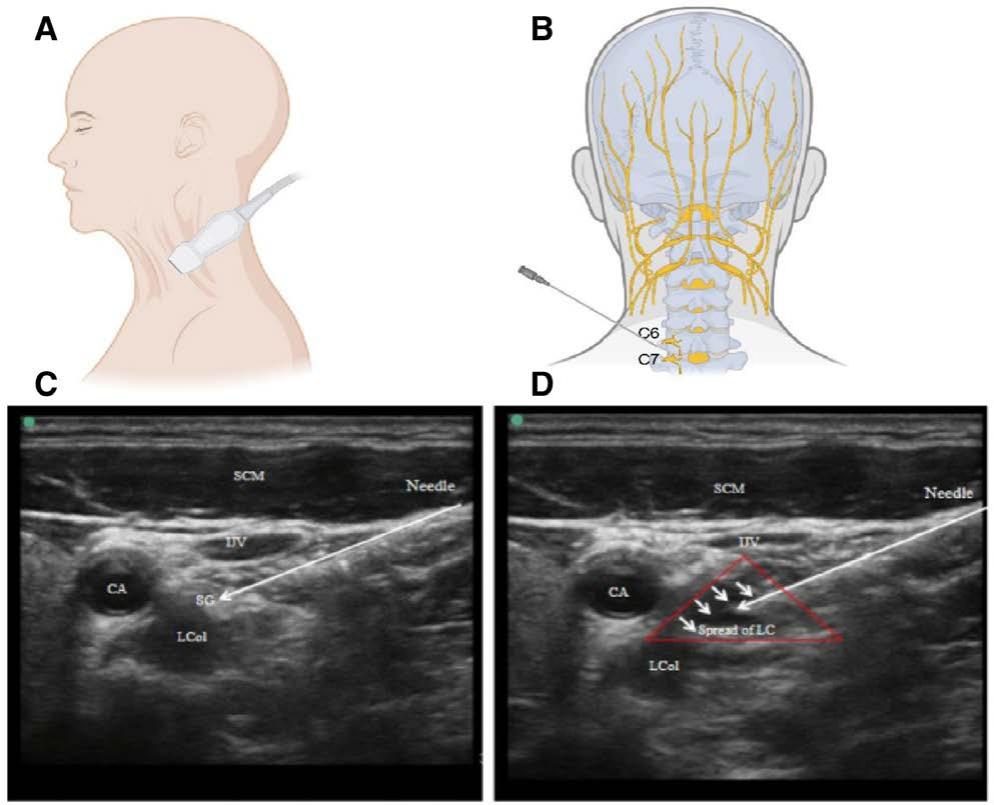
The anatomy and ultrasound image of the stellate ganglion block. (A and B) Anatomy structure and puncture point of stellate ganglion block; (C and D) the ultrasound image of the stellate ganglion block. CA = carotid artery, IJV = internal jungle vein, LC = local anesthetic, Lcol = Longus colli muscle, SCM = sternocleidomastoid muscle.
